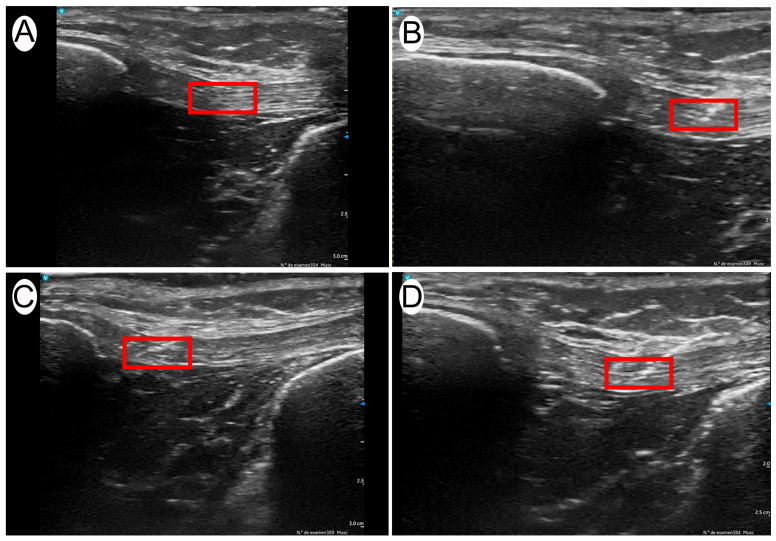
Representative ultrasound images of different percutaneous electrolysis intensities applied for one second: (A) 0.1 mA; (B) 1 mA; (C) 2 mA; (D) 6 mA.
